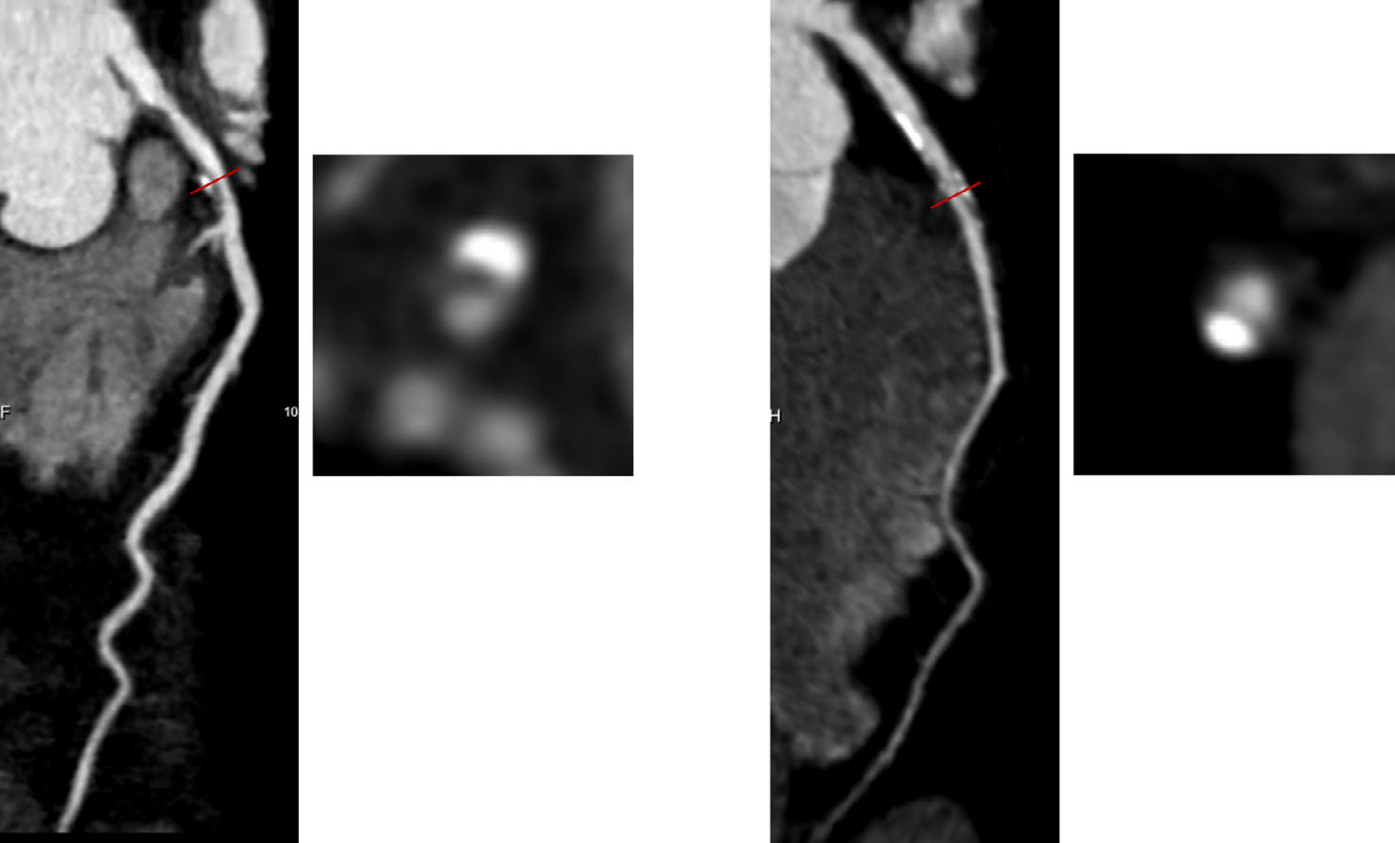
Representative CCTA images. CCTA, coronary computed tomography angiography
